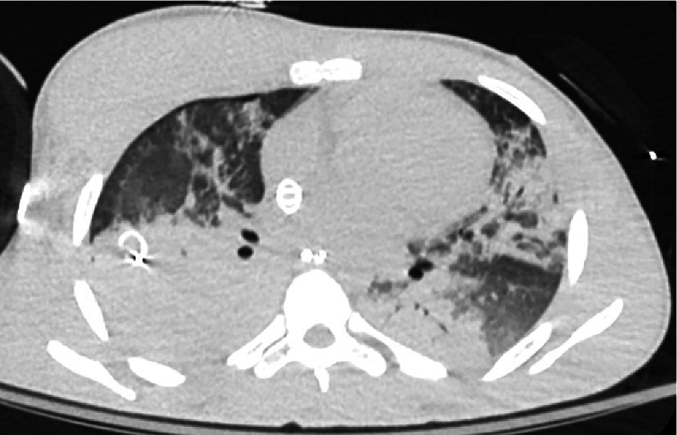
CT chest demonstrating severe pulmonary injury in case 1. Note the extensive bilateral consolidation.
